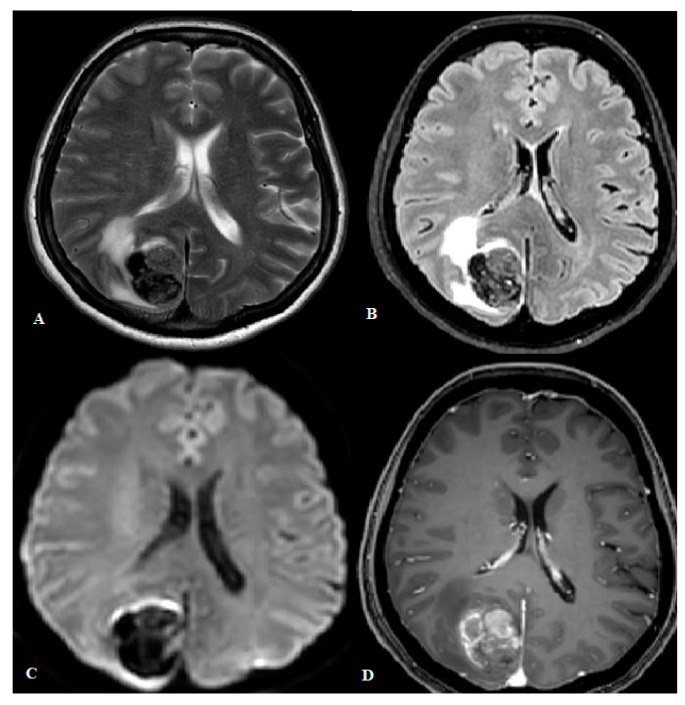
(A–D) Axial T2w (A), FLAIR (B), DWI (C) and T1w post contrast (D) scan of a 67-year-old woman displaying a parietal meningeal melanocytoma.
